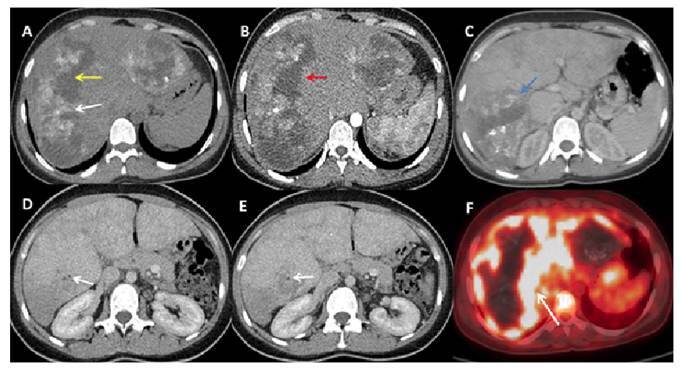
Noncontrast abdominal computed tomography (CT) demonstrates (A) hepatic lesions with peripheral calcifications (white arrow) and central cystic areas (yellow arrow). Peripheral contrast enhancement is observed during the arterial (B, red arrow) and portal venous phases (C, blue arrow). Perfusion reduction secondary to portal vein thrombosis is noted (D, E, white arrow). Positron emission tomography CT shows (F) intense fluorodeoxyglucose uptake around the lesions (white arrow).
